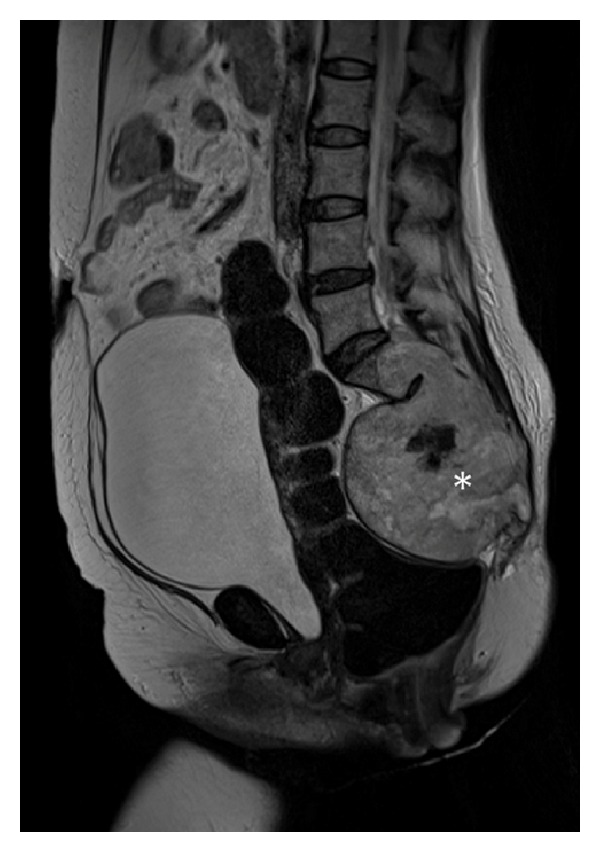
MRI scan of the large chordoma (white star) of the sacrum: T2-weighted sagittal image.
